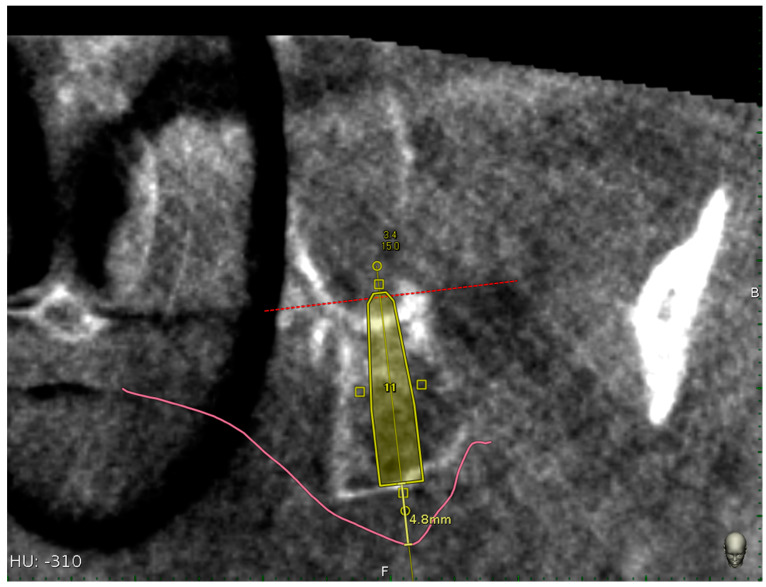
Mucosa thickness linear measurement using the implant centric view of the CBCT.
